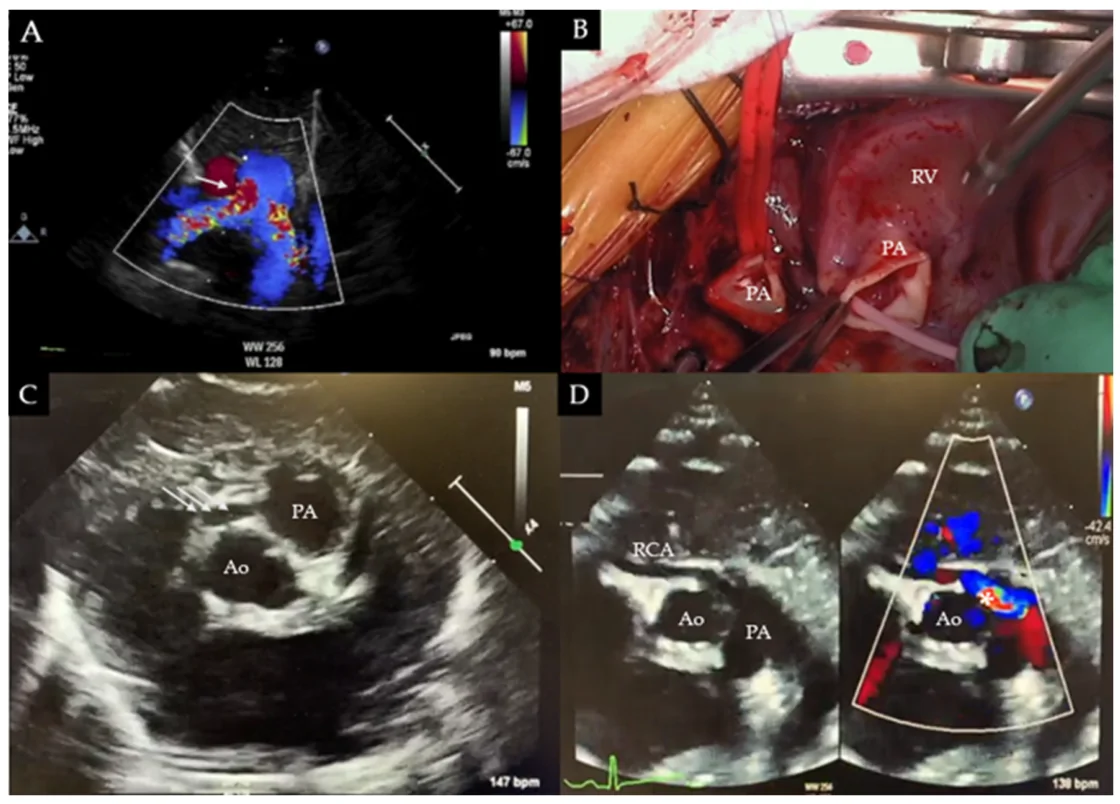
(A–D): Anomalous origin of the coronary artery from the pulmonary artery, (A) preoperative transthoracic echocardiography showing retrograde flow (white arrow) in the main pulmonary artery which is diagnostic, (B) intraoperative photo showing the transected main pulmonary artery (PA) and a probe is inserted in the left coronary artery ostium that is arising from the pulmonary root (ALCAPA), (C) transthoracic echocardiography showing the anomalous right coronary artery arising (multiple white arrows) from the main pulmonary artery (ARCAPA), and (D) retrograde flow (white asterisk) is seen into the pulmonary artery; RV: right ventricle; PA: pulmonary artery; Ao: aorta; RCA: right coronary artery.
